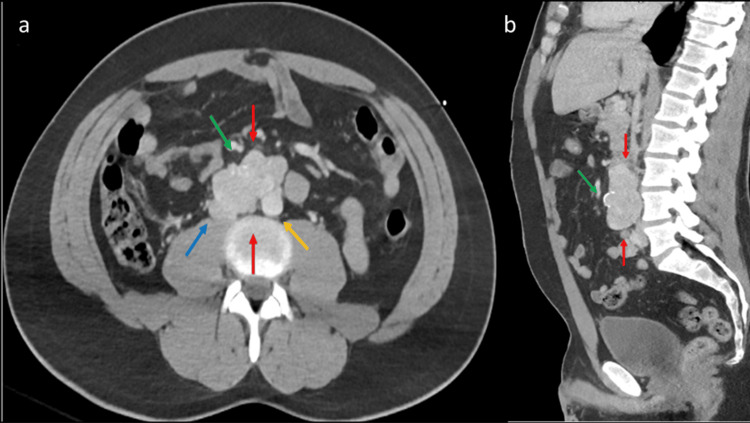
Axial and midsagittal CT of the abdomen and pelvis demonstrating retroperitoneal mass from metastatic paraganglioma. (a) Axial CT of the abdomen and pelvis demonstrates a well-circumscribed multilobulated soft tissue mass within the retroperitoneum (red arrows), intercalated between the aorta (light orange arrow) and inferior vena cava (IVC) (blue arrow). The lesion did not encase vessels and was therefore amenable to surgical resection. Note mild mass effect on the IVC and minimal calcification in the anterior tumor (green arrow). (b) Midsagittal CT of the abdomen and pelvis demonstrates the soft tissue density mass, presumed metastatic lymph node conglomerate from paraganglioma.
